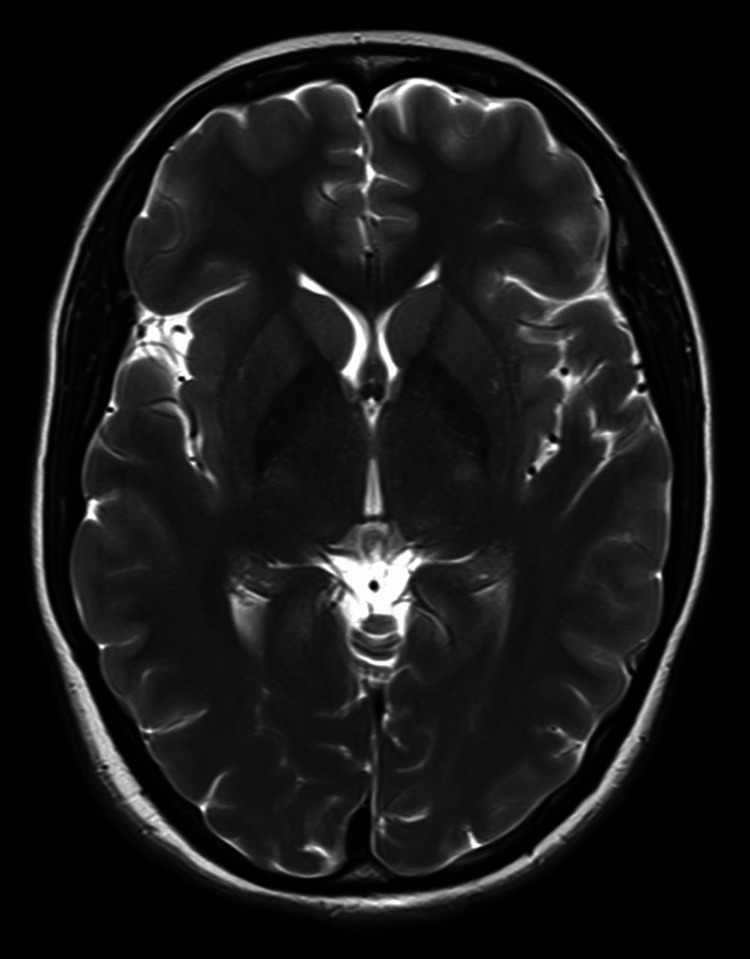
MRI brain with normal results
